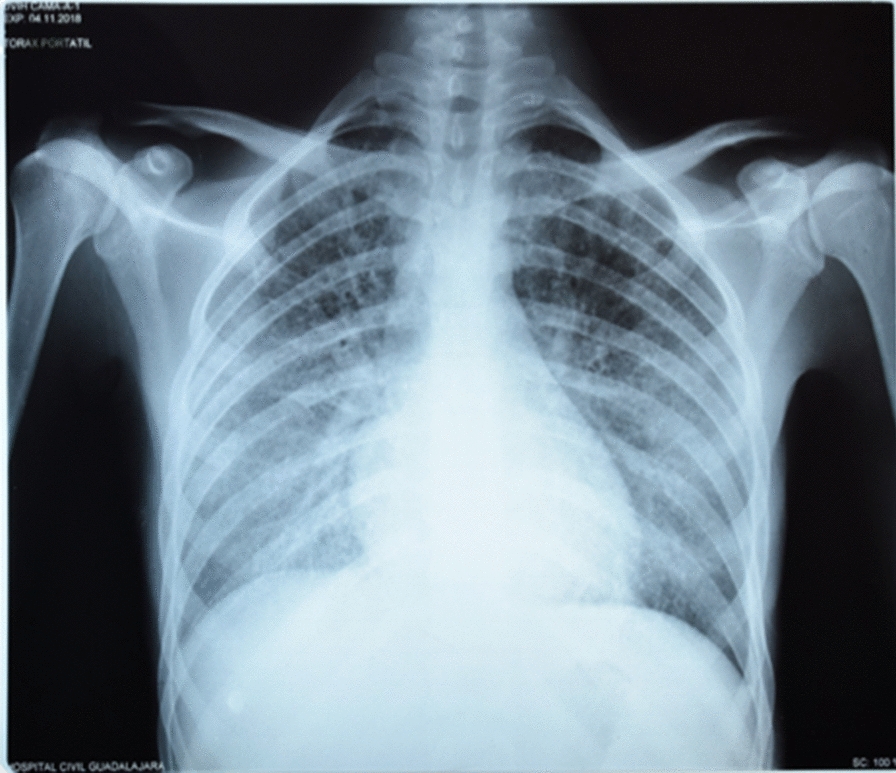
Chest X-ray shows fine bilateral reticulo-interstitial infiltrates
